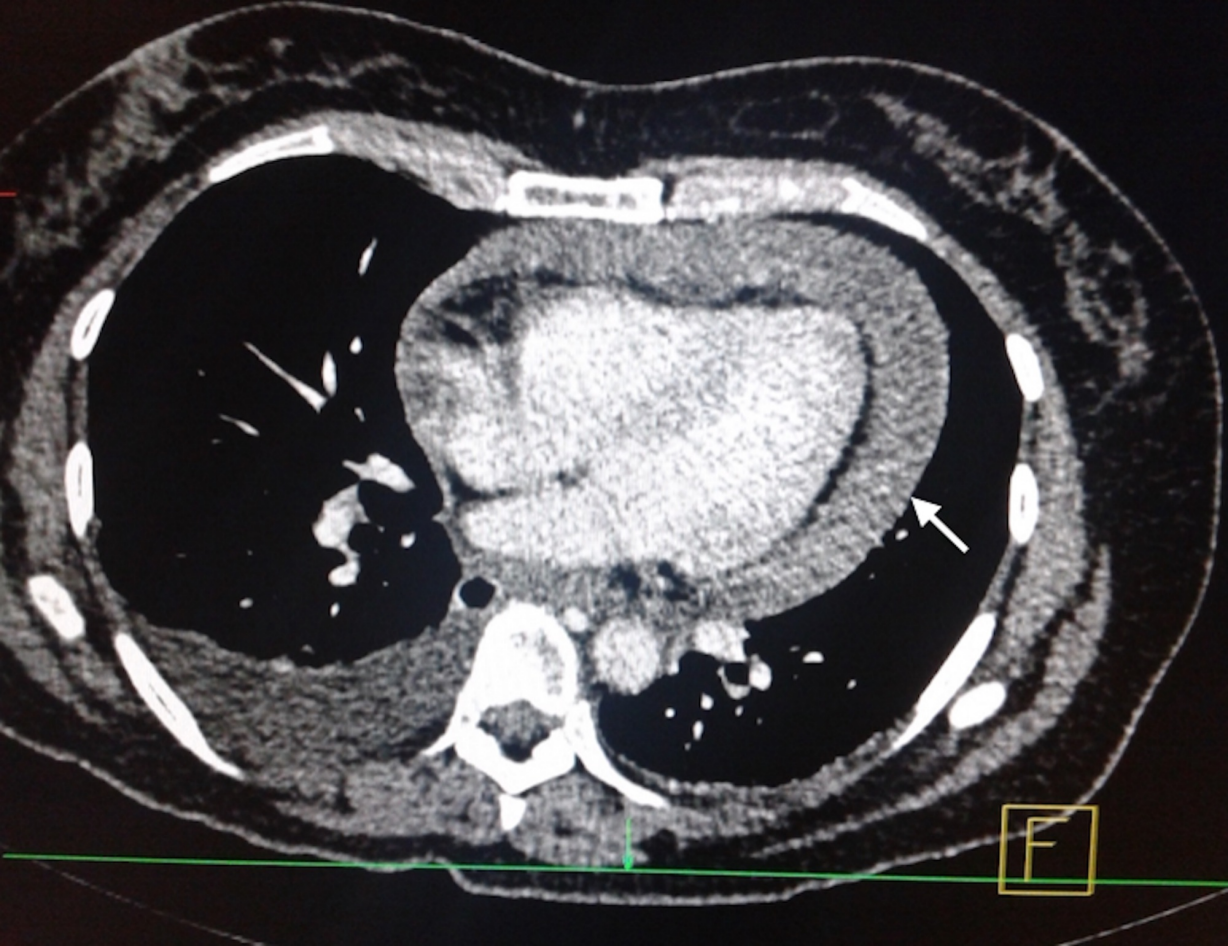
CT scan, axial plane. Pericardial effusion estimated in 400 cc (white arrow).
